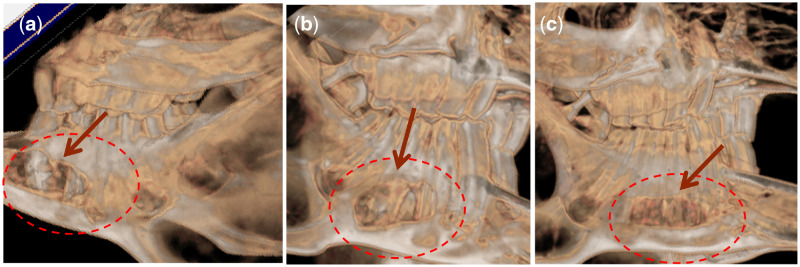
CT Images of mandibular alveolar bone defects implanted with (a) MC, (b) MC/BMP-2, (c) MC/BMP-2/VEGF after 12 weeks.
